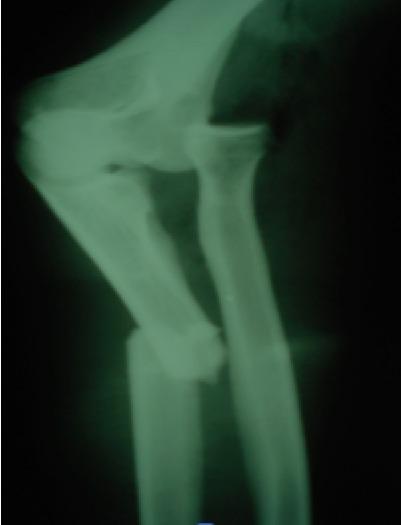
Radiography showing the Monteggia fracture in a second patient
